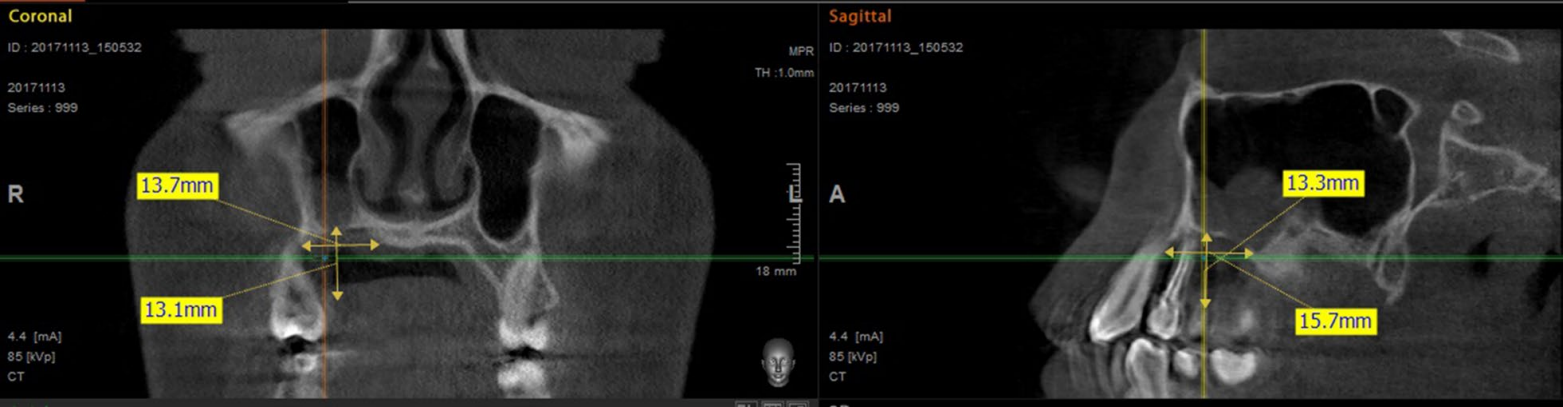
3D computed tomography showing bone defect at 13, 14, 15 teeth region
